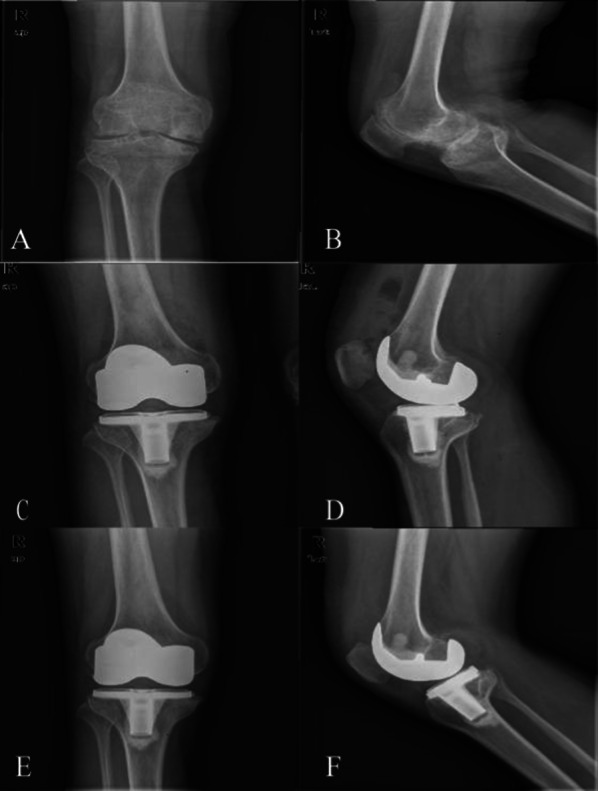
Radiographs of a 35-year-old male patient with severe haemophilic arthropathy. Preoperative X-rays showed extensive destruction of an articular surface, narrowing of the joint space, and a square patella. A Preoperative anteroposterior radiograph. B Preoperative lateral view. C Postoperative anteroposterior radiograph. D Postoperative lateral view. E Final follow-up anteroposterior radiograph. F Final follow-up lateral view
